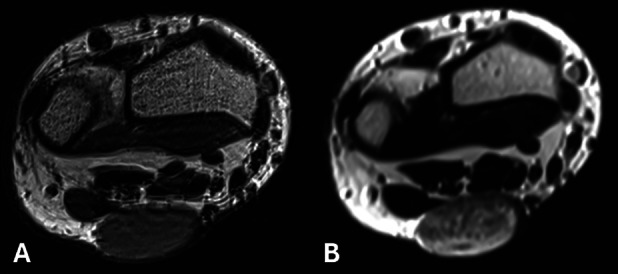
MRI. The lesion is slightly hyperintense compared to muscle on T1-WI and heterogeneous on T2-WI.
